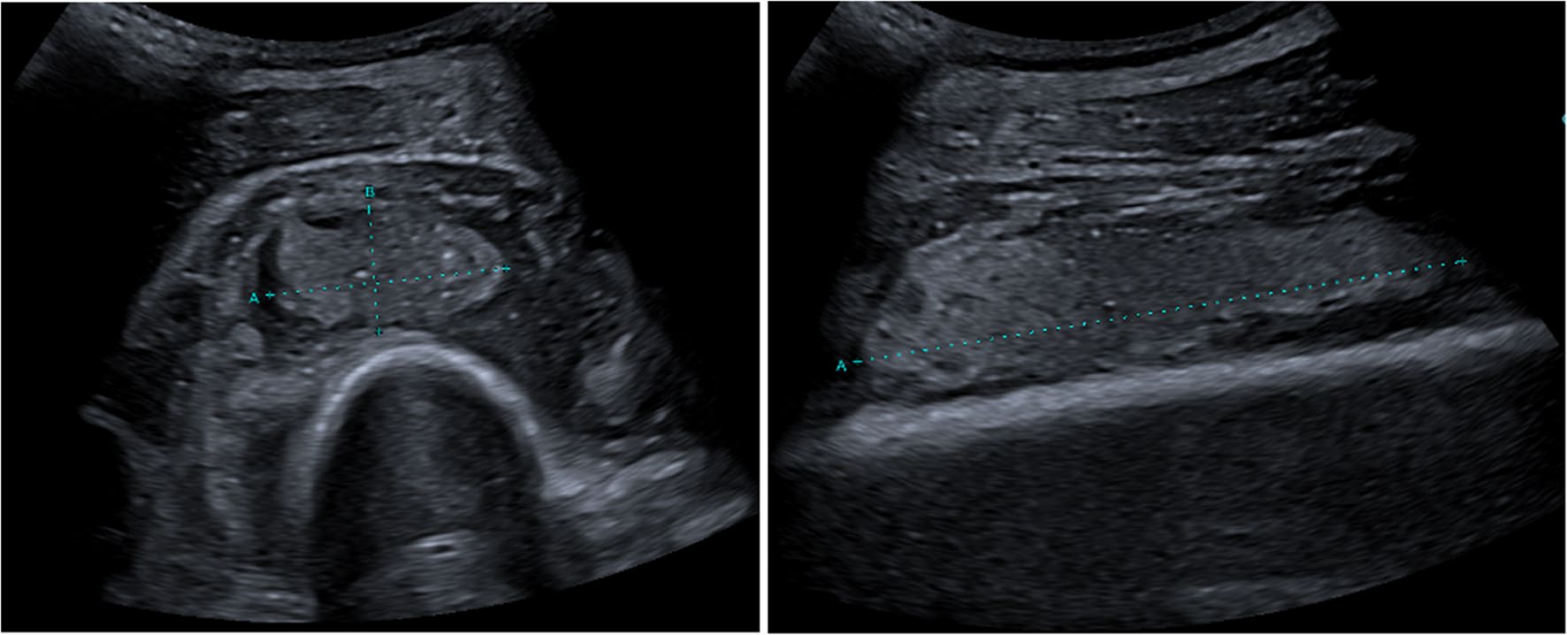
Ultrasound of the left femur showing abscesses near the musculus vastus lateralis (8.0 × 3.3 × 1.7 cm) and musculus intermedius (6.0 × 2.7 × 1.3 cm)
